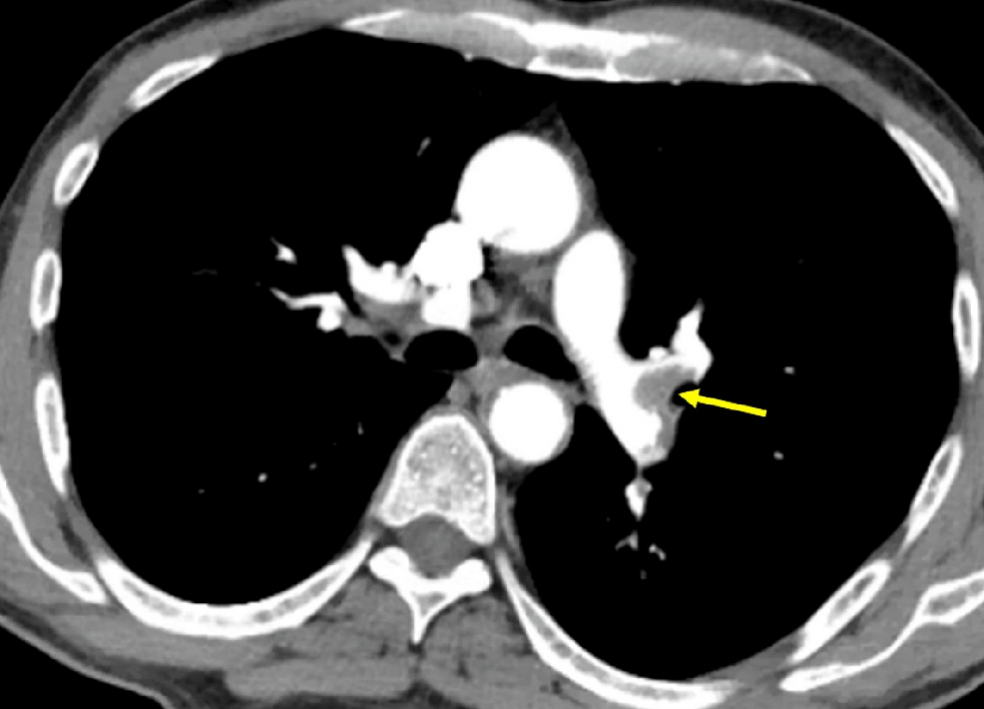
CTPA showing a thrombus in the left main pulmonary artery extending into the left upper and lower lobar branches (yellow arrow). CTPA: computed tomography pulmonary angiography
